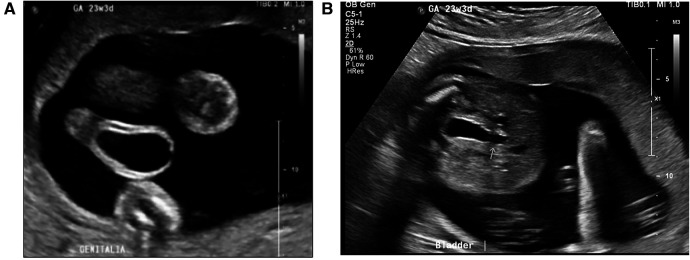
(A) Antenatal ultrasound at 23 + 3 weeks gestation, showing a ventral view of dilated megalourethra. (B) Presence of keyhole sign on the antenatal ultrasound.
